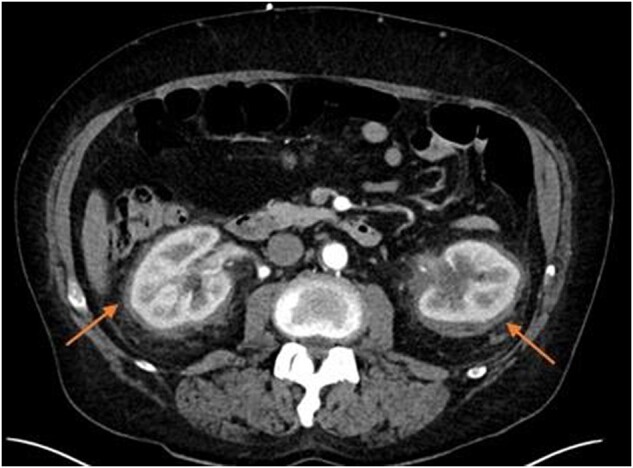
CT of the abdomen showing the typical infiltration of perinephric tissues known as ‘hairy kidney’.
